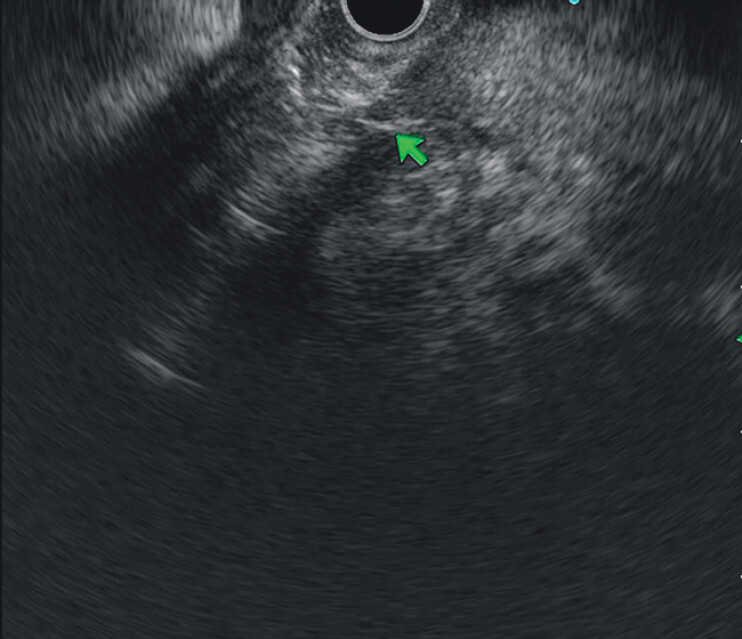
Ultrasound endoscopic image. Before foreign body removal (the green arrow points to the foreign body).
